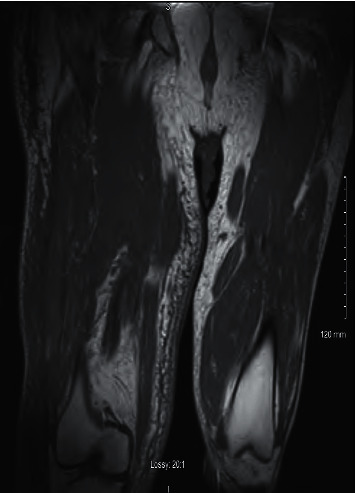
MRI without contrast of the right femur: bilateral inflammatory changes manifested by extensive subcutaneous edema with interfascial free fluid as well as severe myositis. Disproportionate enlargement of the right vastus medialis muscle which is diffusely heterogeneous with a patchy area of intramuscular hemorrhage is shown.
